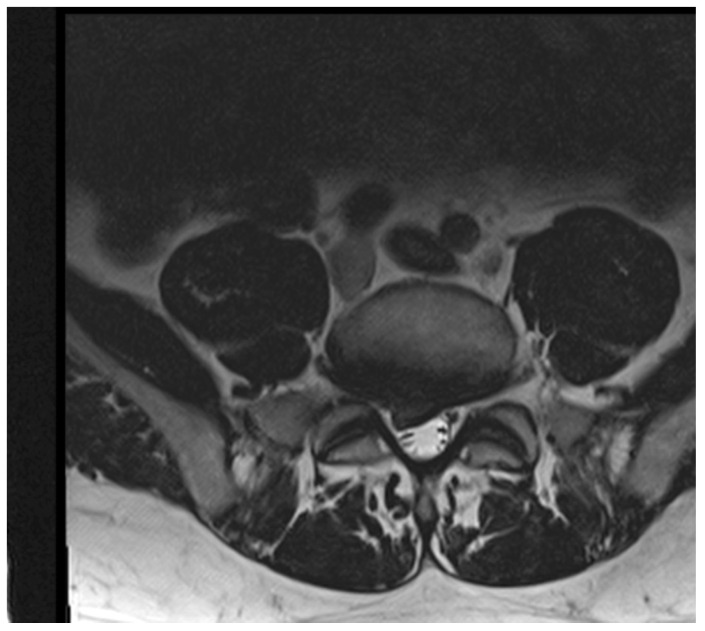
An example image of intervertebral disc extrusion MRI (1.5 T, T2 transverse planes).
